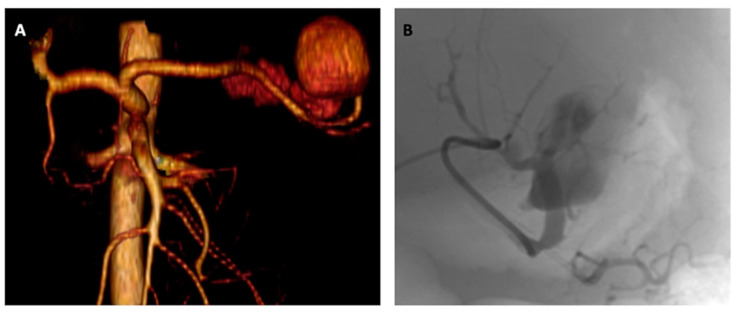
(A) Three-dimensional volume rendering CTA reconstruction showing the huge ruptured splenic artery aneurysm, and (B) intraoperative super-selective digital subtraction angiography confirming the CTA findings.
